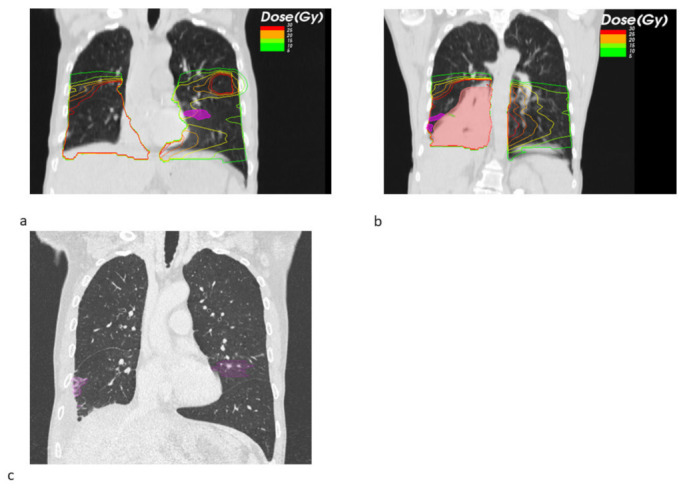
Sagittal radiotherapy (RT) planning CT slices showing the sum EQD2 isodoses and pneumonitis contours (magenta) for a SBRT+ ICB case (a). The same patient received simultaneous contralateral hypofractionated mediastinal RT with original isodoses revealing a relevant lung dose (b). Exemplary sagittal slice from the follow-up CT showing the bilateral pneumonitis and its contours (magenta) (c).
